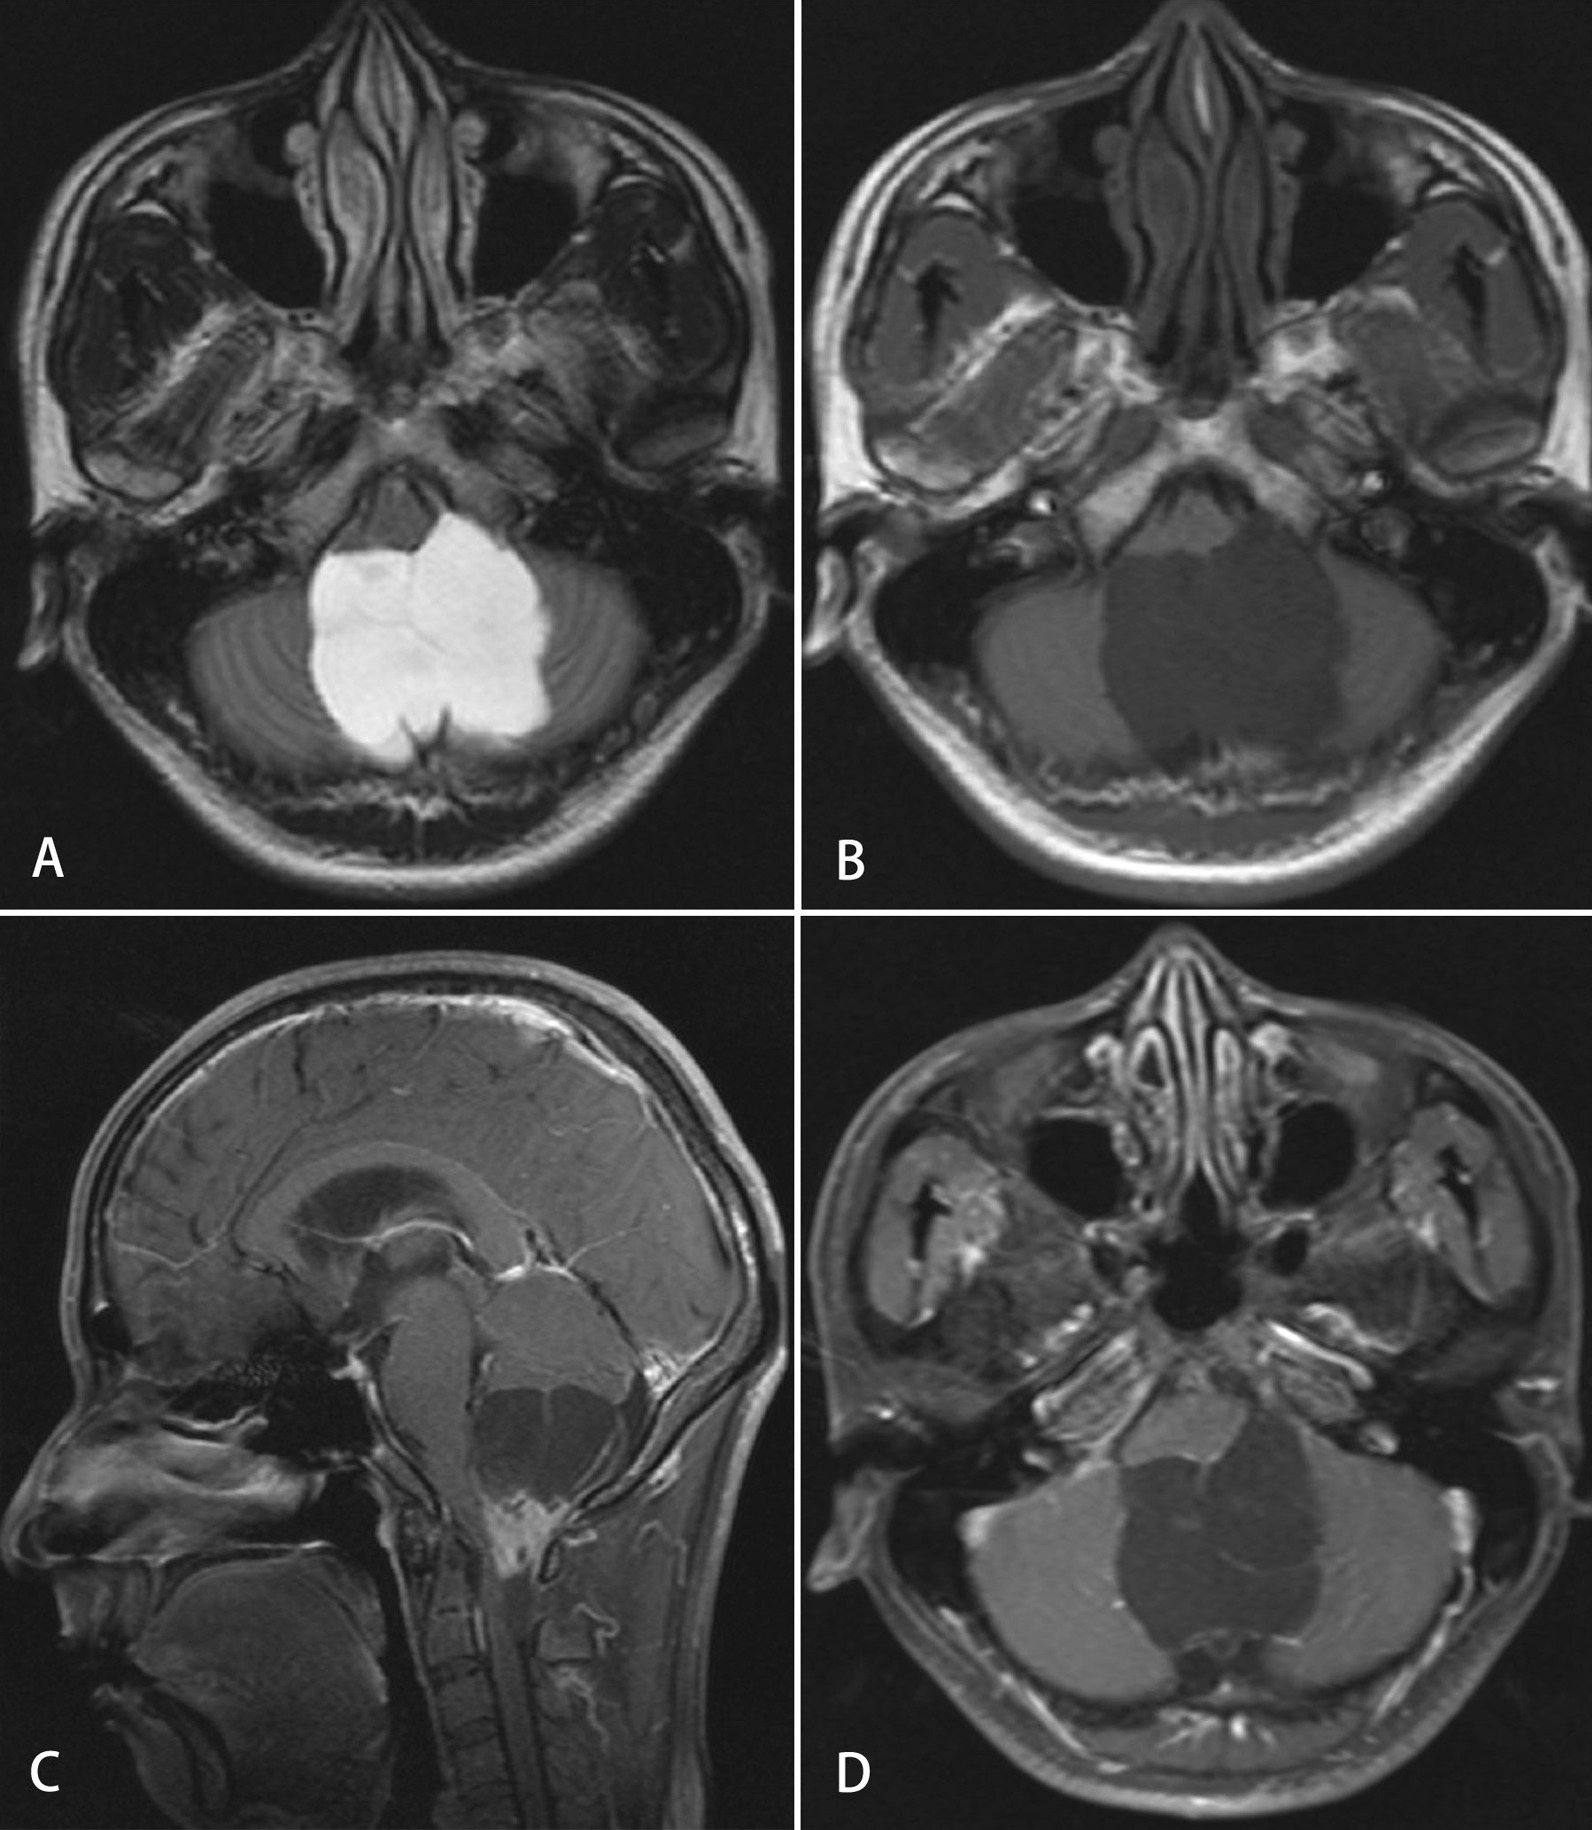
MRI findings of a 27-year-old man with intraparenchymal schwannoma. A well-defined solid and cystic mass with septa could be observed in the vermin of the cerebellum (A, B). The solid components and the septa are enhanced after the contrast agents’ injection (C, D)
